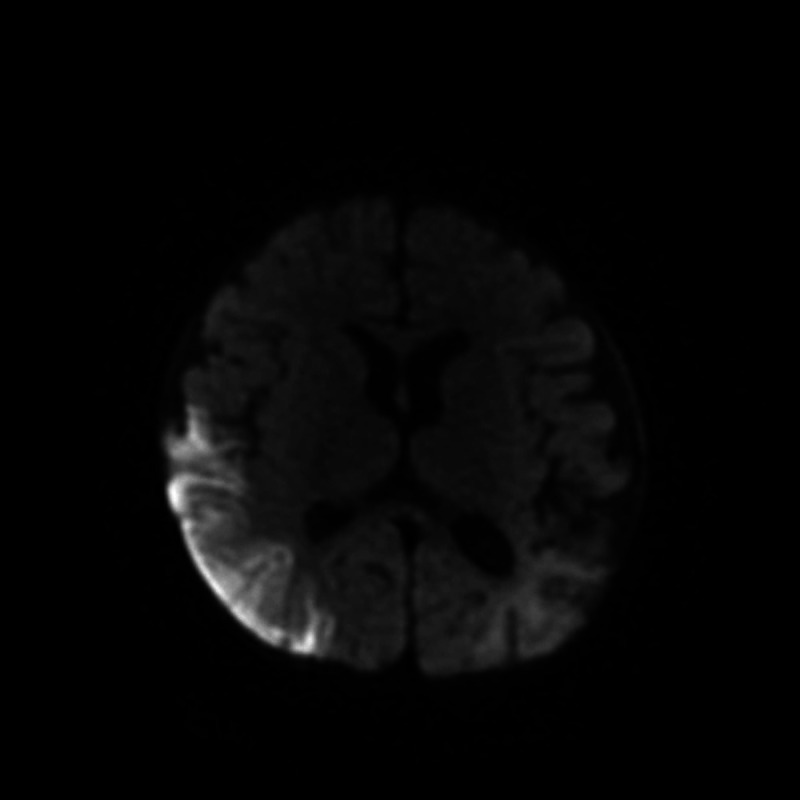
MRI of a 7-month, 3-week old female demonstrating a right-sided parietal lobe infarct.
